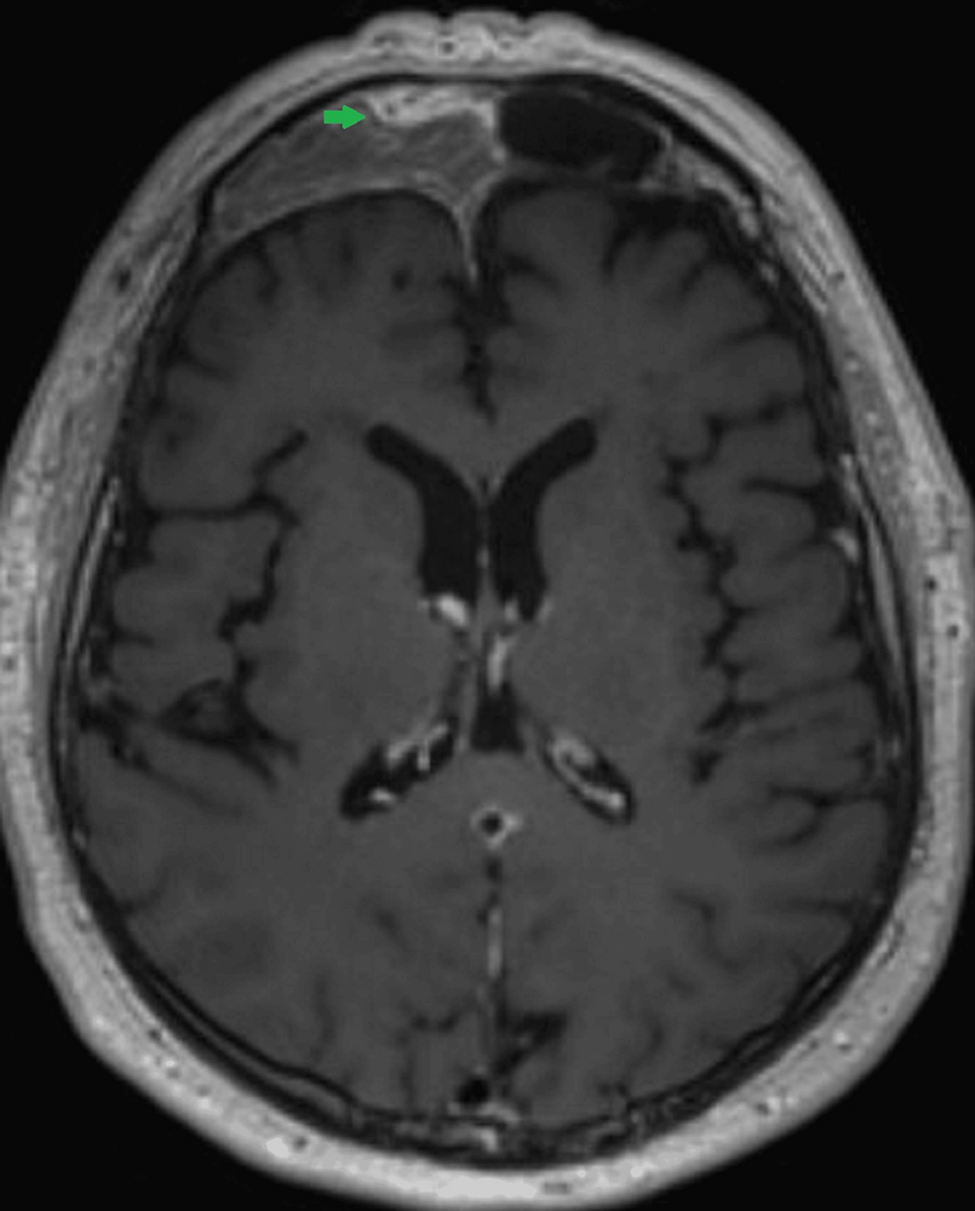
MRI brain (axial, T1 post-contrast) Small residual enhancing tumor in the right frontal sinus (green arrow).
